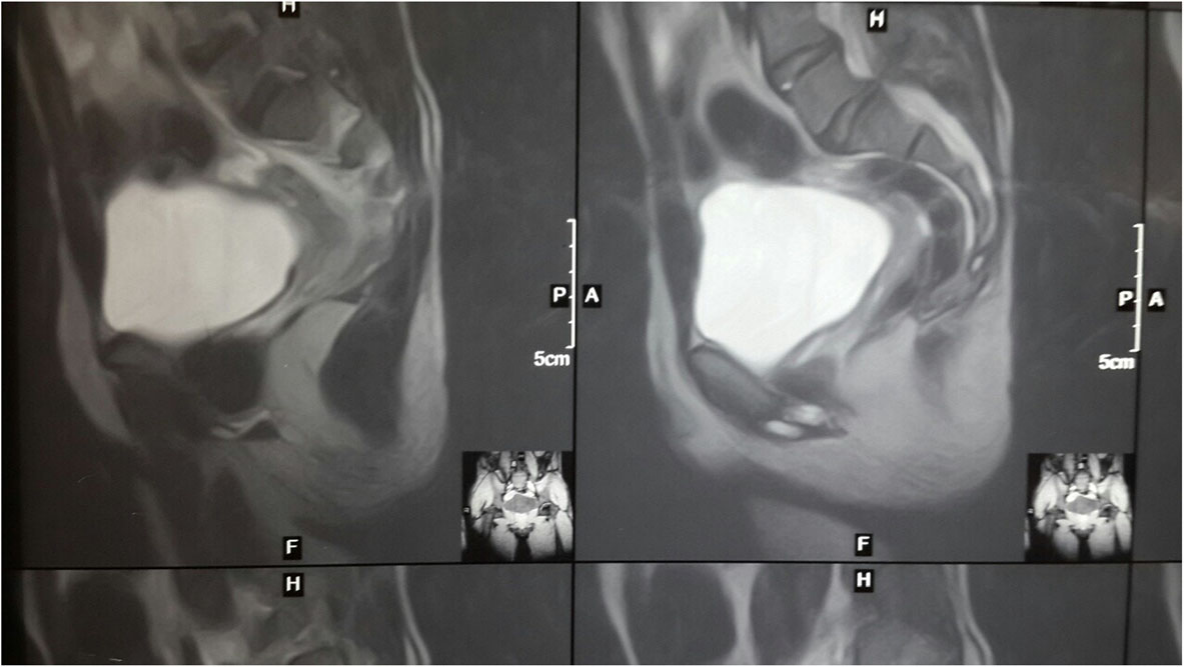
Magnetic resonance imaging of the pelvis showing Müllerian structures
